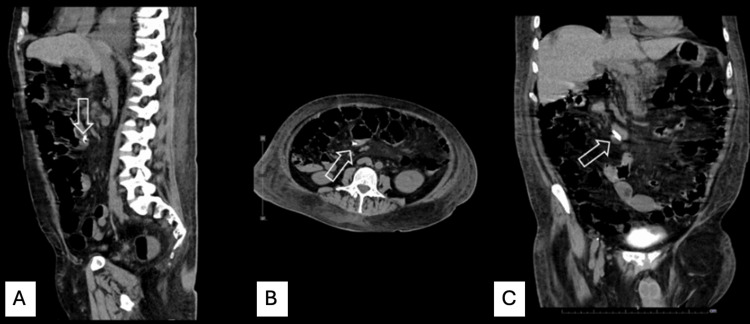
Hemostatic clip Non-contrast CT image (B) and sagittal (A) and coronal (C) reconstructions demonstrating a hyperdense, irregular structure (white arrow) compatible with a hemostatic clip.
